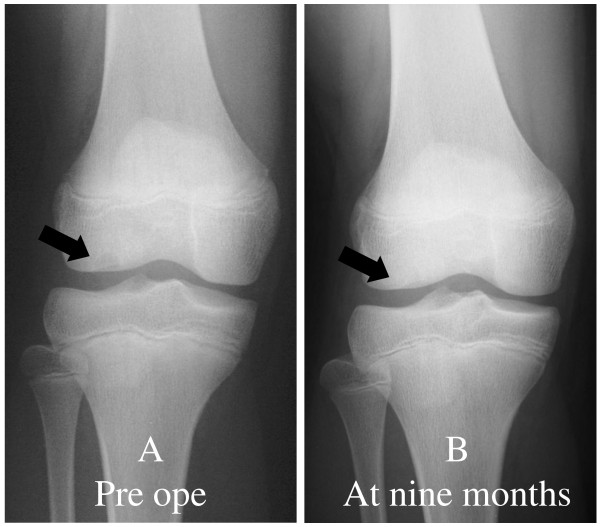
Pre- and postoperative radiographs. (A) Preoperative radiograph showing a small circumscribed radiolucency with a thin sclerotic margin in the subchondral region of the lateral femoral condyle (black arrow). (B) Radiograph taken at nine months showing healing of the lesion.
